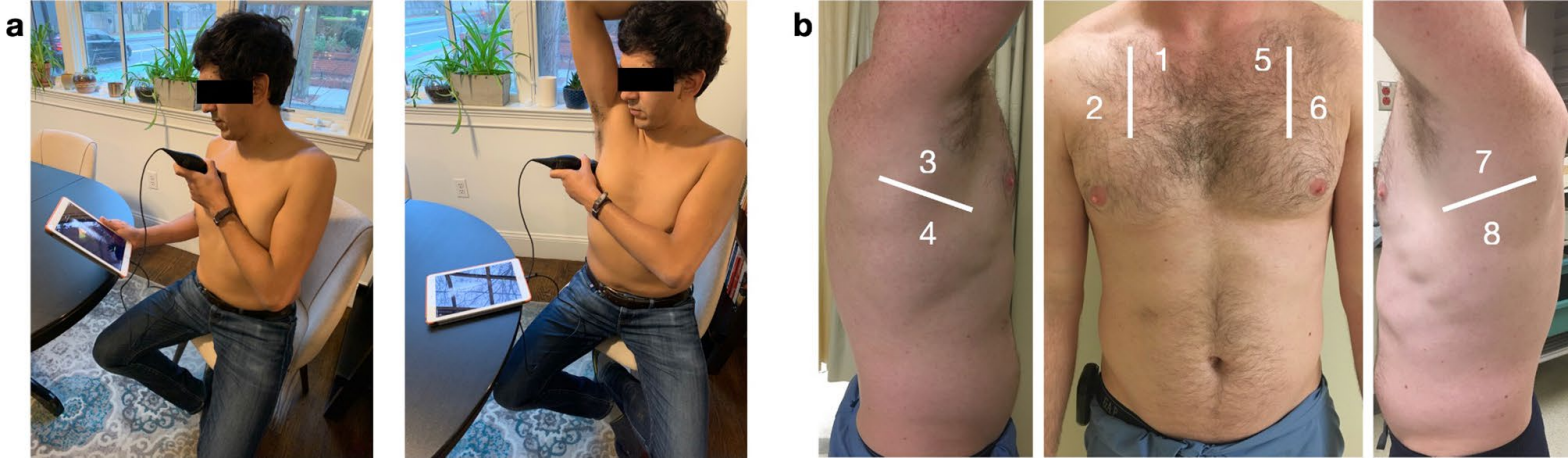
Novice-performed lung ultrasound scanning protocol. (A) A novice self-performs a LUS using a hand-held ultrasound probe attached to an iOS device. (B) 8-zone scanning protocol performed by both novices- and expert-sonographers.
